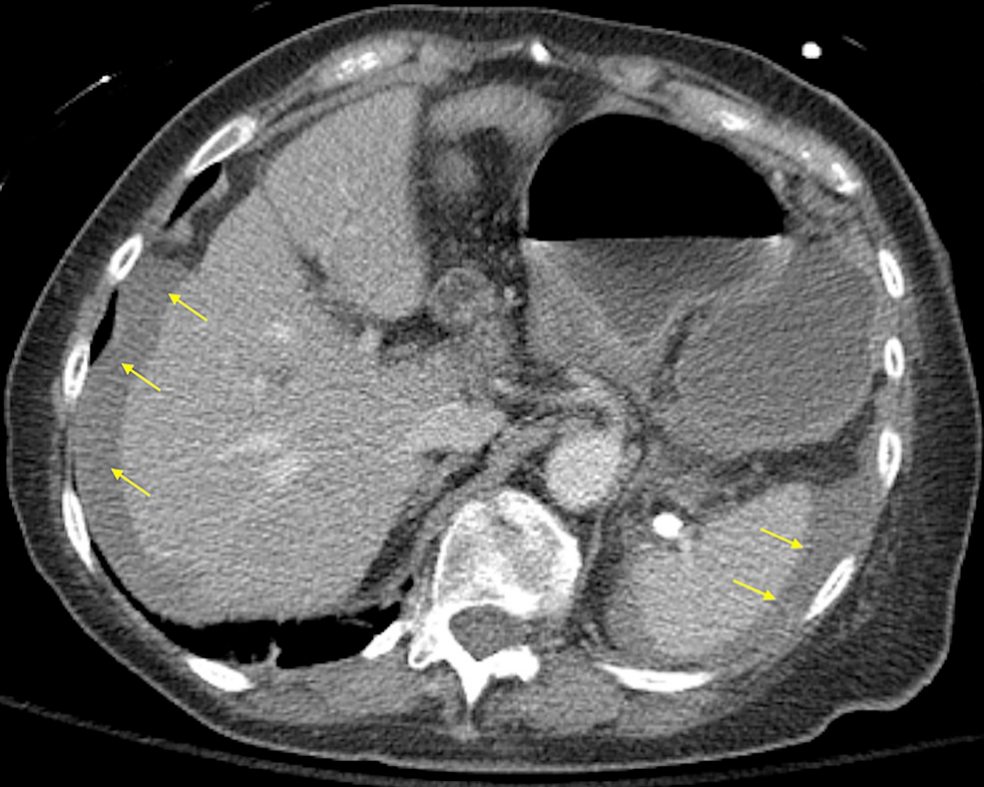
CT with contrast angiography with an axial view of a large hiatal hernia with suspected organo-axial volvulus and free fluid surrounding the distal esophagus with moderate hemoperitoneum surrounding the stomach, liver, and spleen (yellow arrows)
